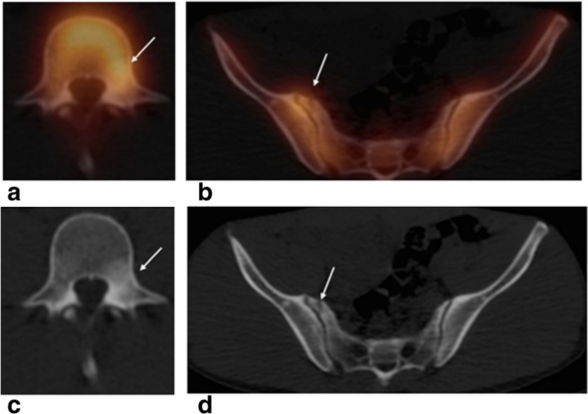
Pedicle stress reaction in an 18-year-old male wrestler complaining of left-sided back pain. Incidental finding of a small old avulsion fracture of the right sacral ala at the joint capsule insertion a Axial fused SPECT/CT image shows mildly increased uptake at the left L4 pedicle, which on axial CT image (c) is associated with sclerosis in the left pedicle region. b Fused SPECT/CT axial image of the sacrum shows minimal uptake associated with a small avulsion fracture at the right sacral ala on axial CT image (d)
